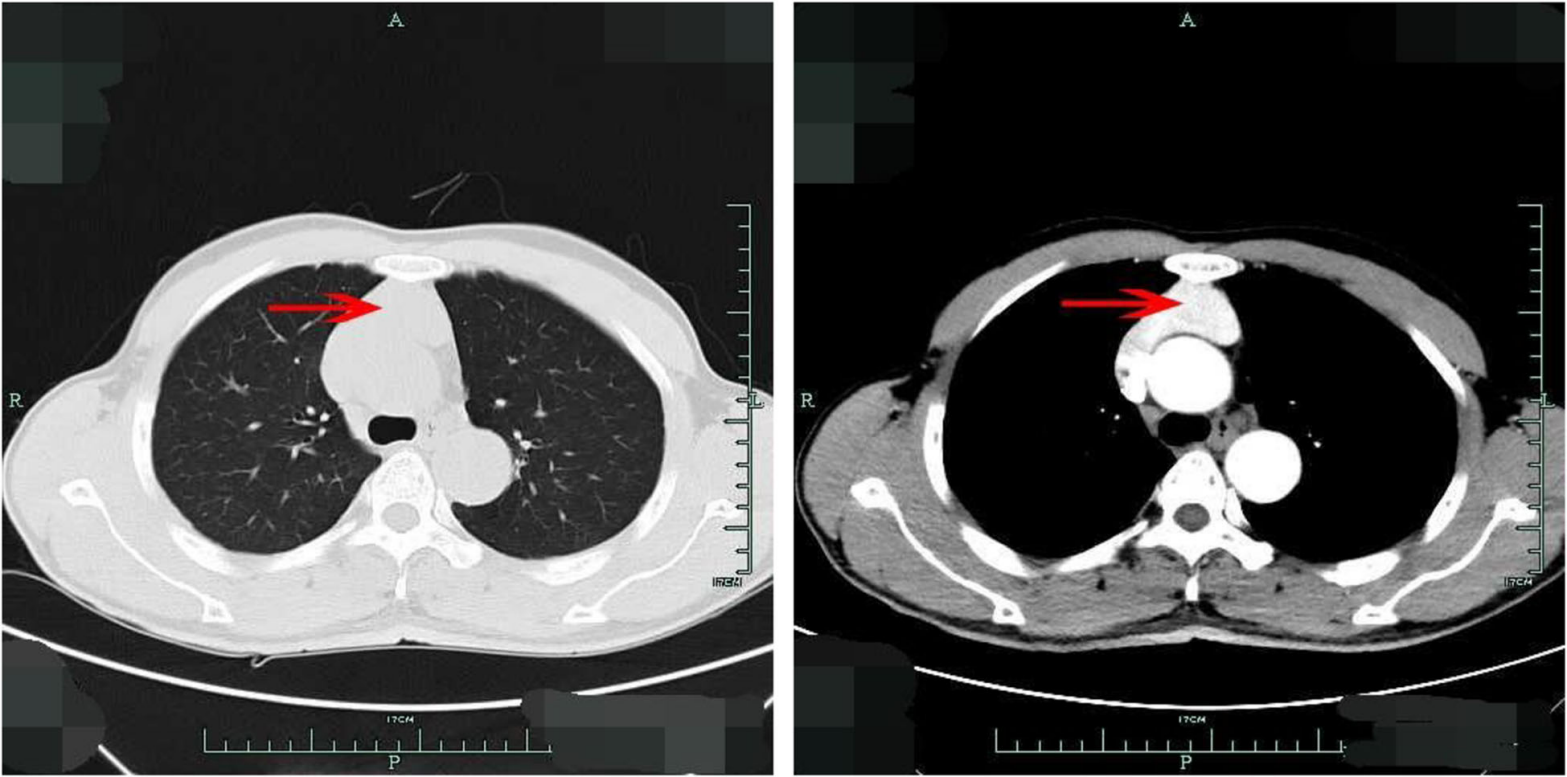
Computer tomography examination of the patient. A large mass of anterior mediastinum was found in CT examination (red arrow), which was enhanced in the artery phase
